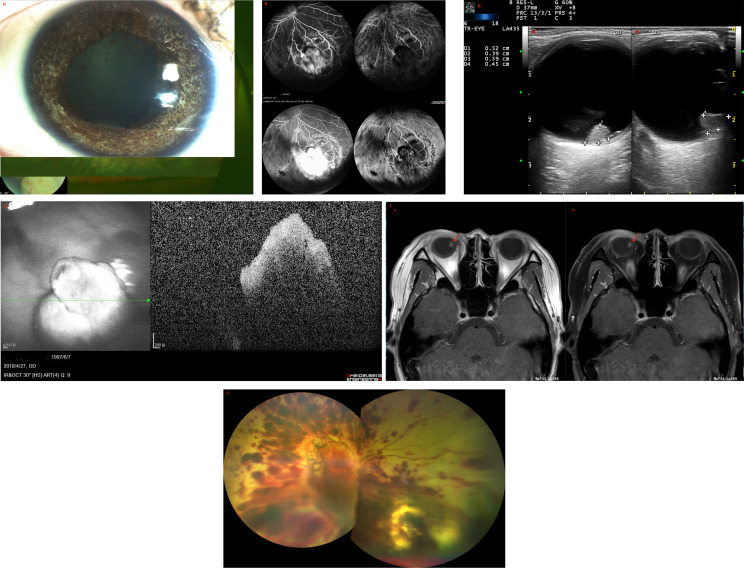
(A) Fundus examination of the right eye of patient 1 revealed a large white dome-shaped retinal tumor in the inferonasal quadrant with feeding vessels and a few vitreous cellularities. (B) On fundus fluorescein angiography (FA), multiple areas of the tumor displayed mottled hyperfluorescence in the early phase and hyperfluorescence with intense leakage in the late phase. On indocyanine green angiography (ICGA), the tumor showed hypofluorescence during all stages. (C) Color Doppler imaging (CDI) revealed a pedunculated mass with inconsistent reflectivity of moderate-intensity and no choroidal excavation, as well as arterial blood signals in the tumor. The size of the elevated lesion was 5.2 × 3.9 × 5.9 mm^3^. (D) Optical coherence tomography (OCT) revealed that the tumor had a sloped dome-shaped elevation, with a hyperreflective anterior surface and vitreous seeds, and normal macular fovea. (E) MRI revealed that the tumor was far from the optic nerve in the right globe, demonstrating a slightly higher hyperintensity (arrow) than vitreous in (a) axial T1-weighted MRI, hypointensity (arrow) in (b) axial T2-weighted MRI, and moderate enhancement of the tumor in axial contrast-enhanced T1-weighted, fat-saturated MRI. (F) At the last follow-up 15 months after the last combination treatment, the retinoblastoma demonstrated complete regression into a partially calcified scar. (G) One day after IV-Melphalan, intravitreal melphalan-hemorrhagic retinopathy toxicity occurred. (H) The patient showed signs of iris atrophy and eventually developed phthisis bulbi.
